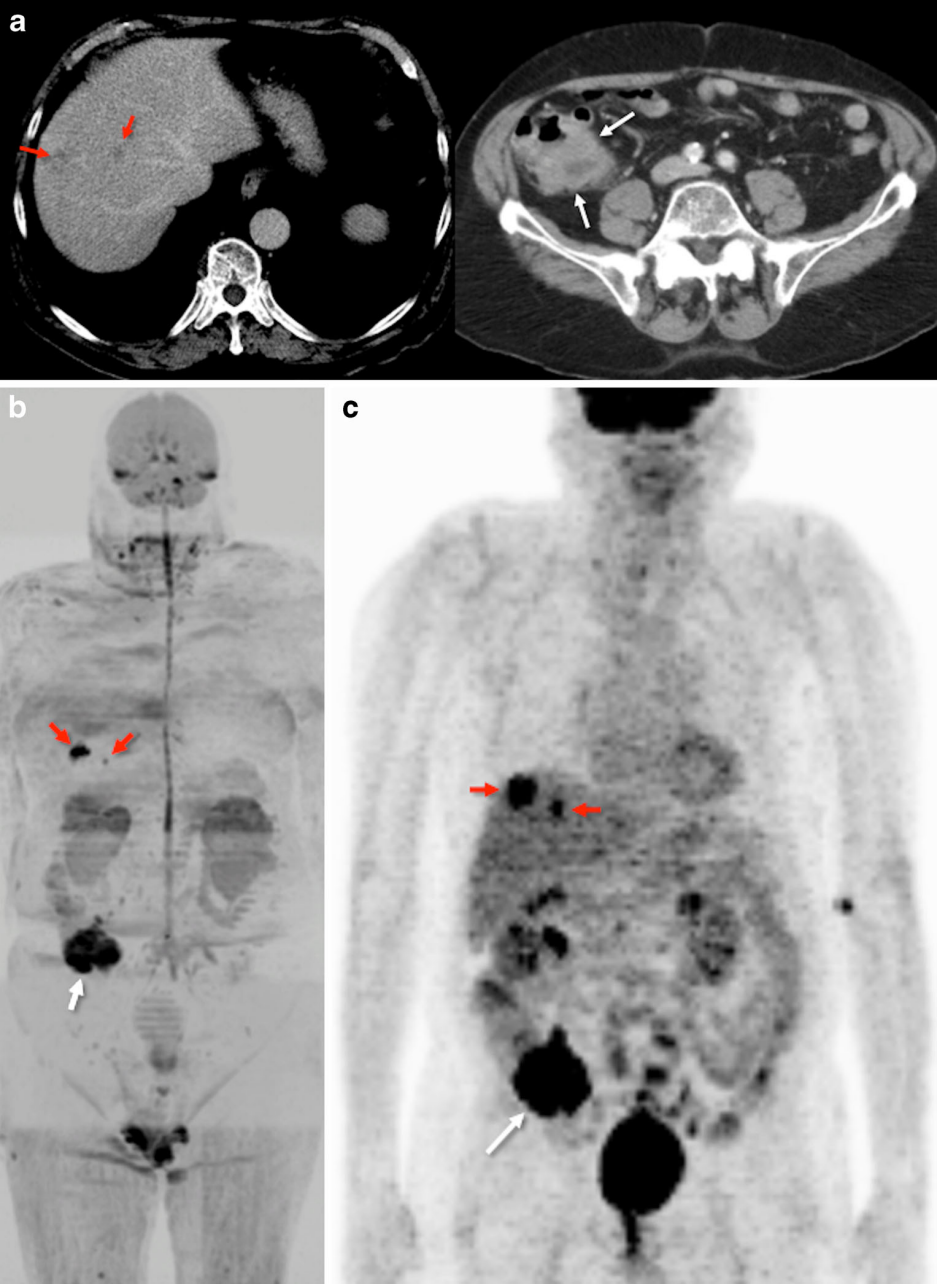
Whole-body diffusion imaging for tumour staging. Conventional portal-phase contrast-enhanced images (a) demonstrated two liver metastases (left – red arrows) and a tumour in the cecum (right - white arrow). Whole-body-diffusion-weighted image with inverted gray scale (b) depicted both focal liver lesions (red arrows) and the tumour in the cecum (white arrow) that showed restriction of diffusion. ^18^F-FDG-PET image (c) evidenced similar findings
